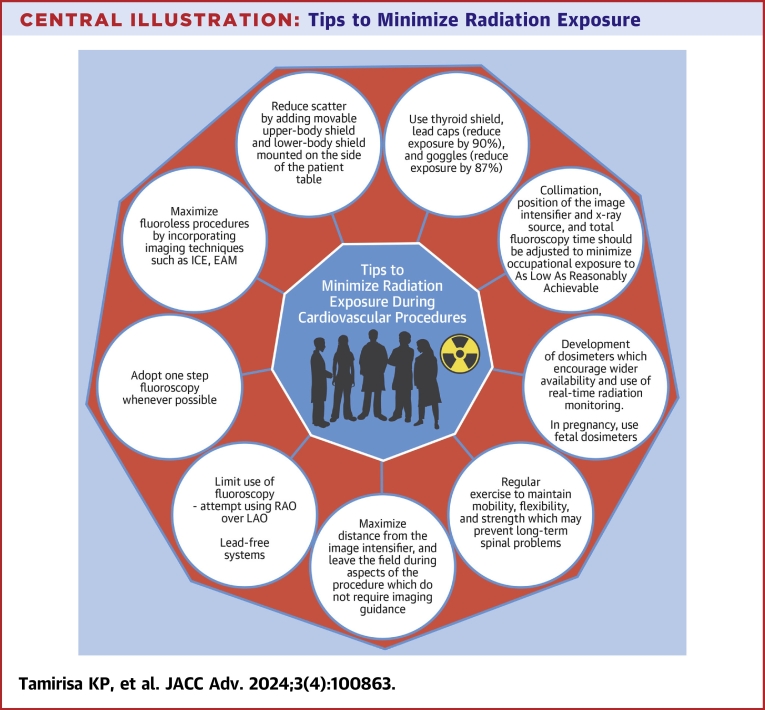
Tips to Minimize Radiation Exposure EAM = electroanatomic mapping; ICE = intracardiac echocardiography; LAO = left anterior oblique; RAO = right anterior oblique.
